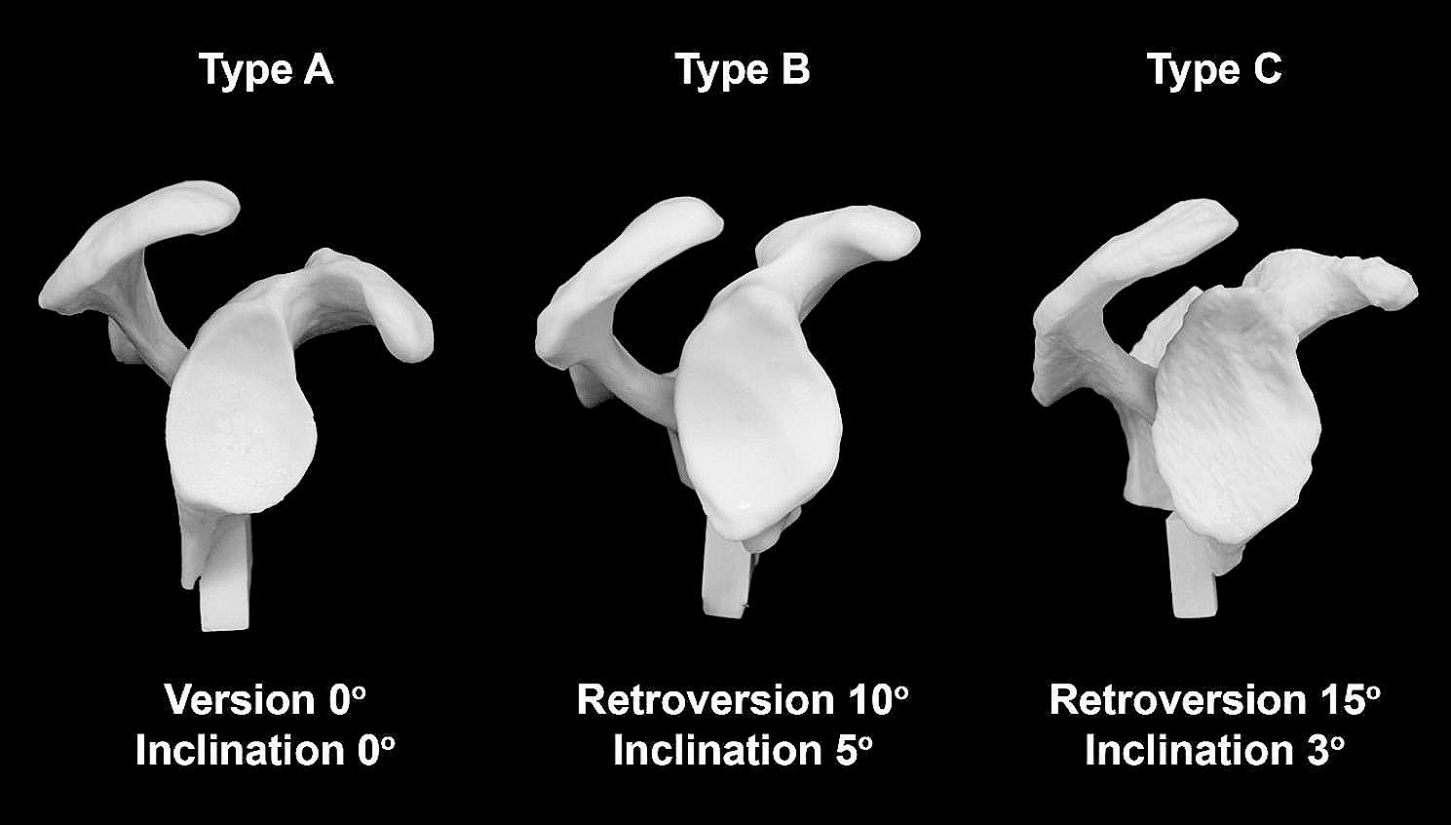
Visualization of different glenoid cast models type A, B and C according to the classification of Walch et al. [10] based on clinical computed tomography (CT) data with planned correction angles for retroversion and inclination
